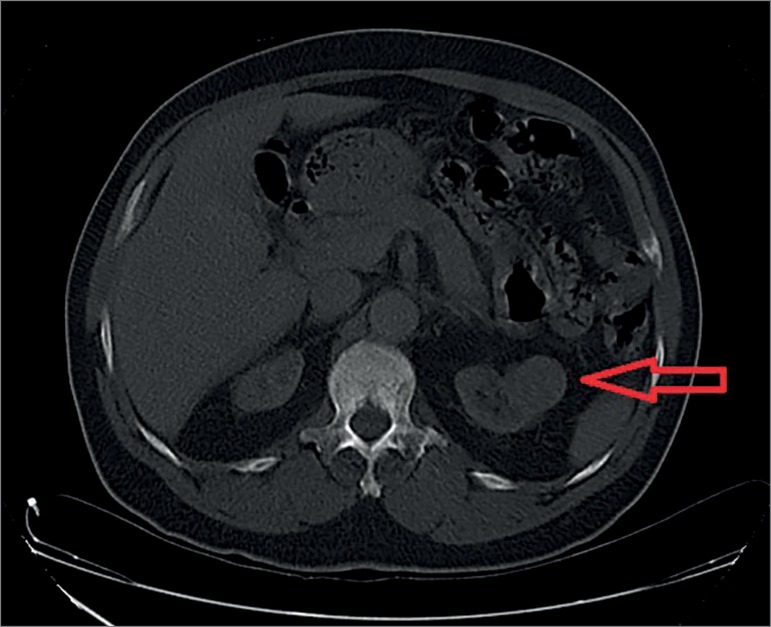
Renal oncocytoma - CT scan exhibiting the typical imaging morphology
